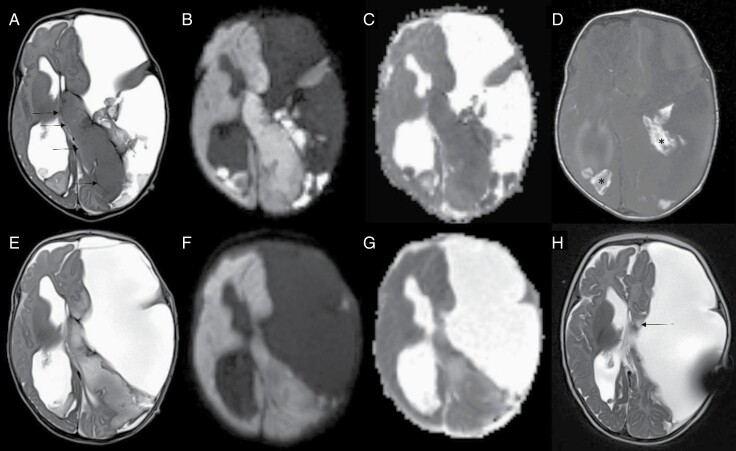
(A–D) First MRI. (A) Axial T2-weighted image shows homogeneous tumor tissue, mainly involving the left frontal and temporal lobe (black arrows), which is of isointense signal intensity compared with the cerebral cortex. Massive posthemorrhagic enlargement of the subdural space is visible on the left. (B and C) Diffusion-weighted images show a slight diffusion restriction as a sign of high cellularity. (D) T1-weighted image shows clots of methemoglobin (*). (E–G) Follow-up MRI at day 24. (E) T2-weighted image shows a substantial increase in the signal intensity of the tumor. (F and G) Diffusion-weighted images show decreasing diffusion restriction. (G) Tumor tissue is now partially hyperintense on apparent diffusion coefficient (ADC) compared with brain parenchyma. Imaging changes in E–G were interpreted as a change from high to lower cellularity. (H) At 5 months after diagnosis, only small, stable tumor residues could be found (black arrow).
